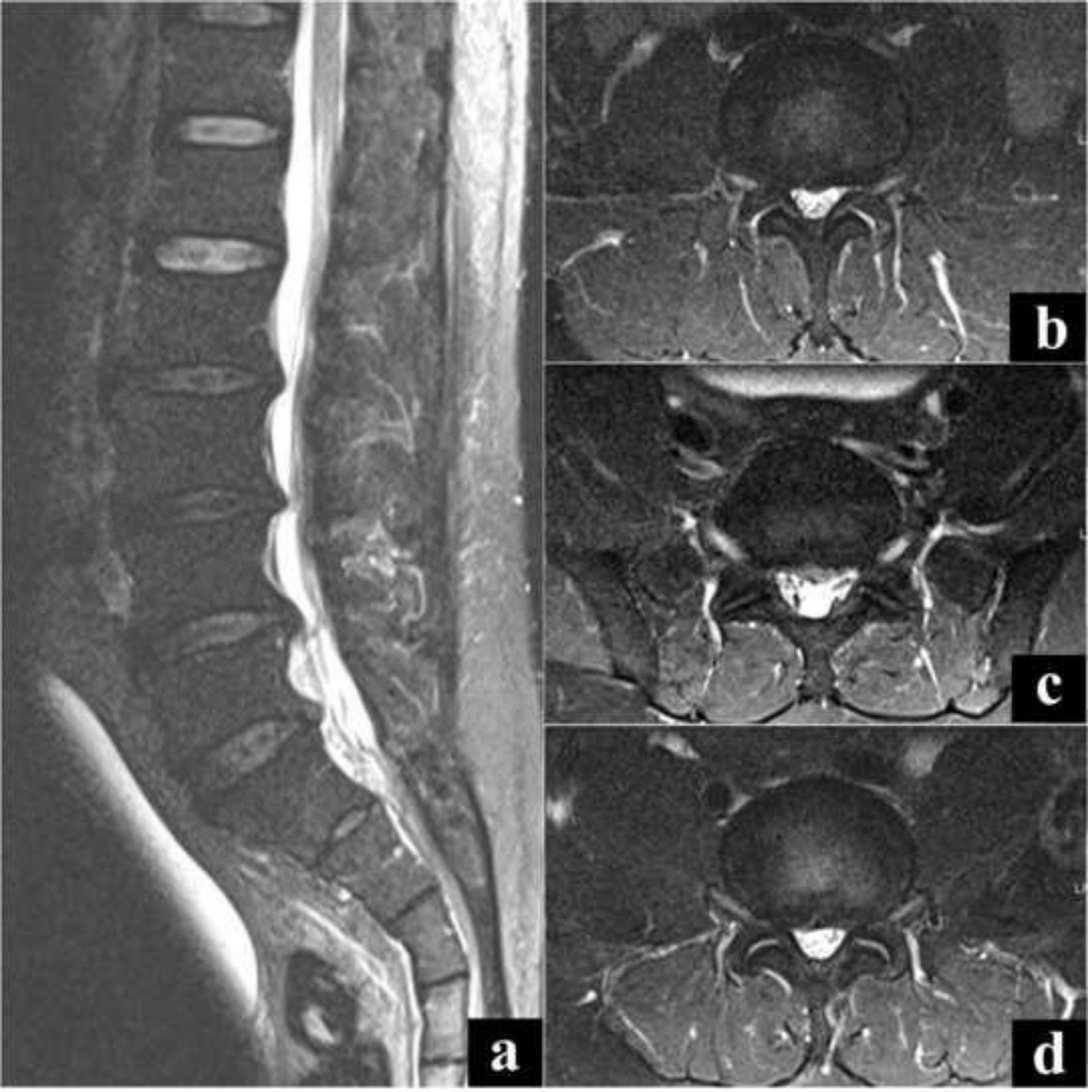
MR image showing multilevel degeneration involving L2-S1. a) Sagittal cut image, b) Axial cut at L2-L3 level, c) Axial cut at L3-L4 level, d) Axial cut at L4-L5 level.
